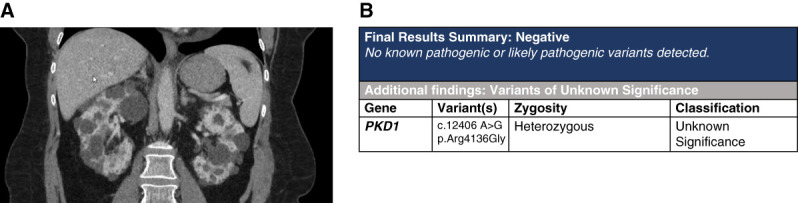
Imaging and genetic sequencing results of case 1. (A) Coronal CT of the abdomen with contrast. The right kidney measures approximately 18.3×10.6×7.6 cm. The left kidney measures approximately 16.9×8.7×6 cm. Both kidneys contain innumerable cysts. (B) Sequencing result report shows negative for relevant pathogenic genes and VUS in *PKD1*. CT, computed tomography.
